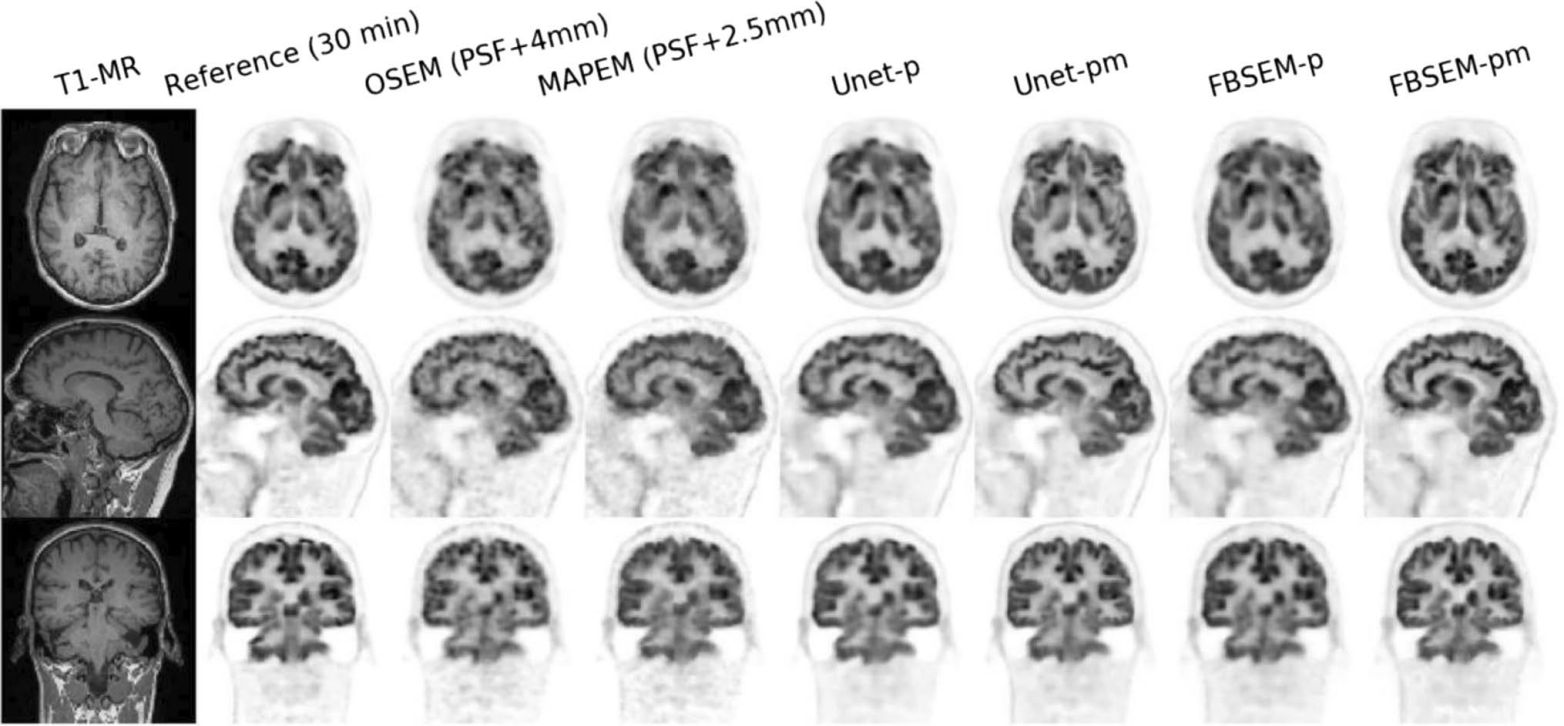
Reconstructed results of the FBSEM-Net [199]. The -p and -pm in the methods represent the use of PET and MRI data for input, respectively. © 2021 IEEE. Reprinted with permission from Mehranian and Reader [199]
